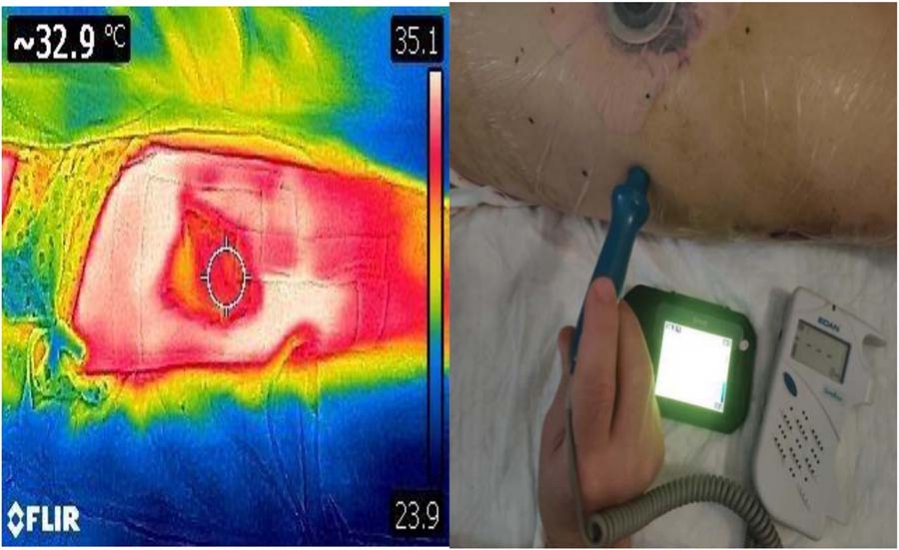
DDT identification of the perforating vessels of the lateral thigh with corresponding confirmation using audio Doppler.
